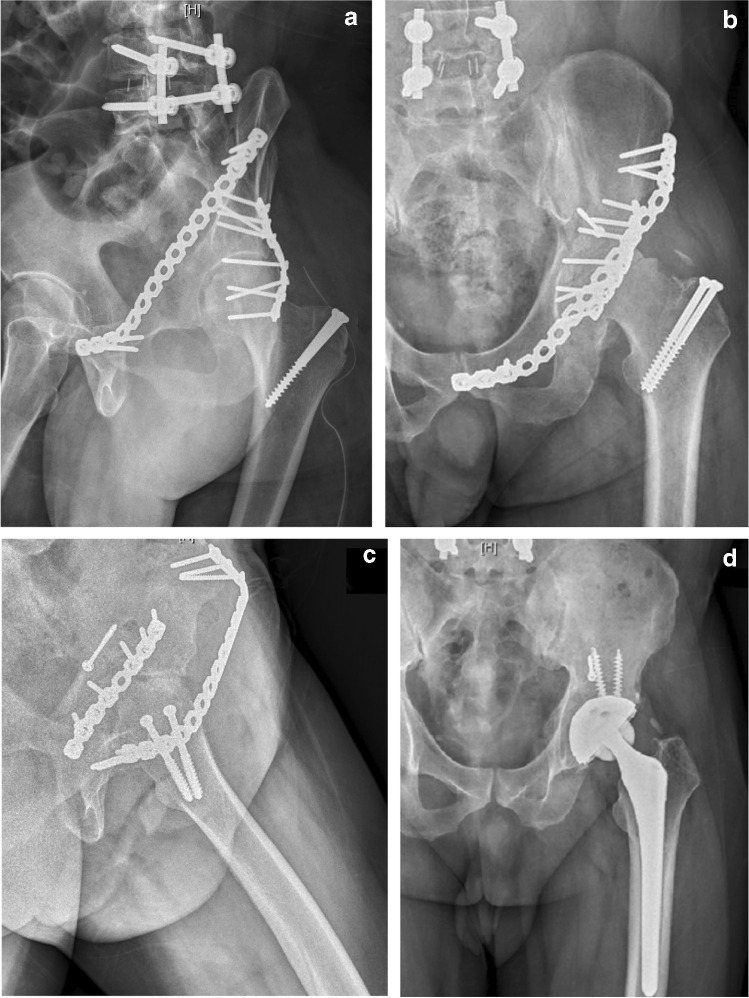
a Post-operative Judet view radiographs of a 52-year-old man (no. 7) showing reduction of left ilium wing, left acetabular (two-column) central complex fracture with dislocated left hip joint and split femoral head fracture. b Pelvis AP and Judet view radiographs after 3 months of surgery showing signs of avascular necrosis and subcapital fracture. e AP radiograph after total hip replacement and removal of SAPB showing excellent radiological outcome but fair functional outcome
